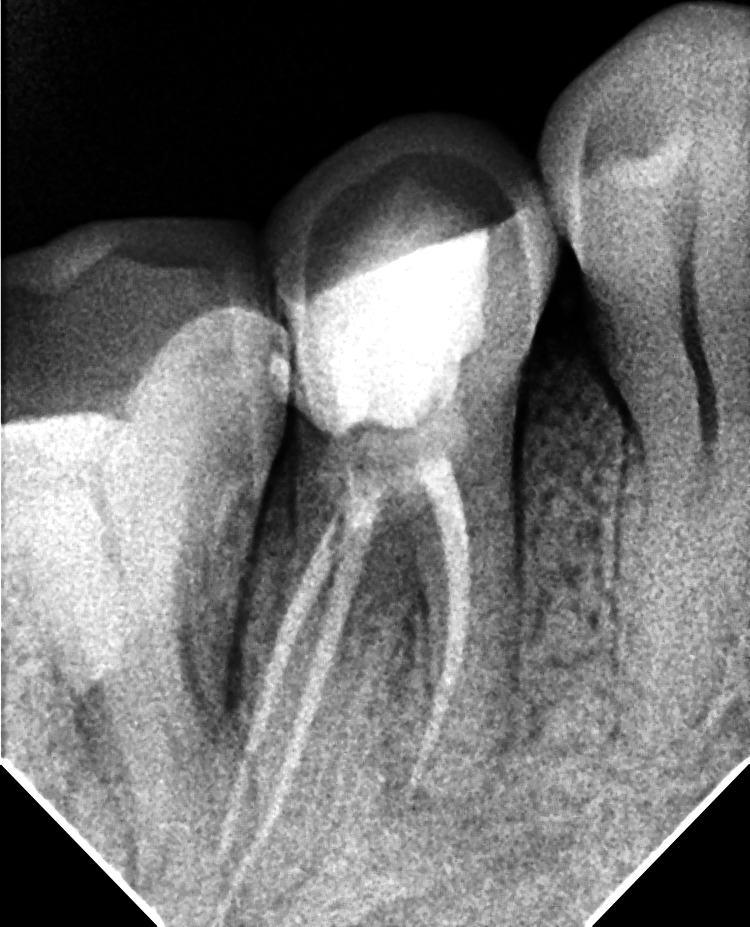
Post-obturation radiograph of mandibular right second premolar of case 1
